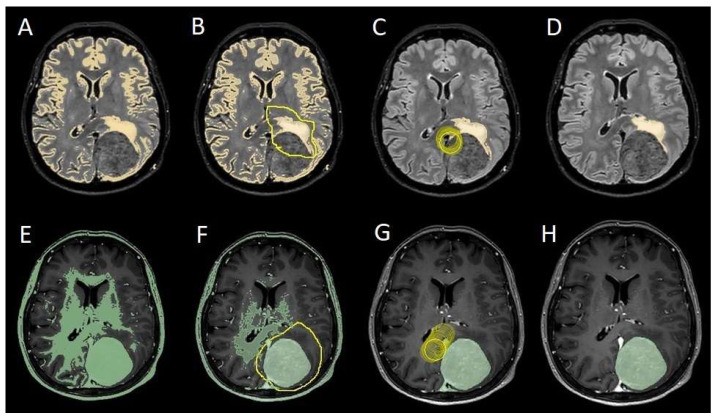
Example of segmentation process with the Slices website. (A,E) Automatic thresholding technique to identify initial regions of interest based on intensity values, respectively, for the PE on the FLAIR sequence and the meningioma on the T1-weighted-with-gadolinium sequence. (B,F) Partially automatic erasure of redundant signal with “erase outside” tool. (C,G) Definition of boundaries of edema and tumor through manual erasure. (D,H) Final segmented volumes. FLARI: fluid-attenuated inversion recovery; PE: peritumoral edema.
